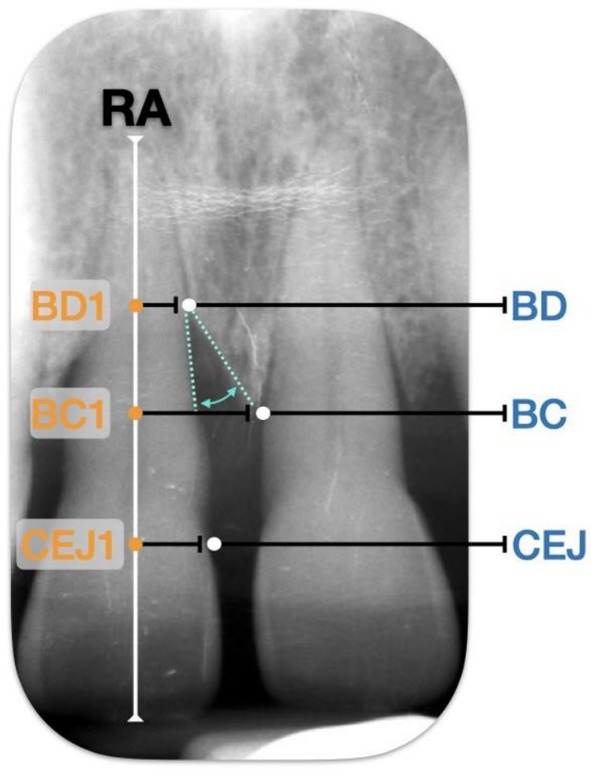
Example of the landmarks used for radiographic measurements. BC, bone crest (most coronal portion of the intrabony defect); BC1, projection of BC on the long axis of the tooth; BD, defect bottom (most apical portion of the intrabony defect); BD1, projection of BD on the long axis of the tooth; CEJ, cementoenamel junction; CEJ1, projection of CEJ on the long axis of the tooth; RA, root apex.
